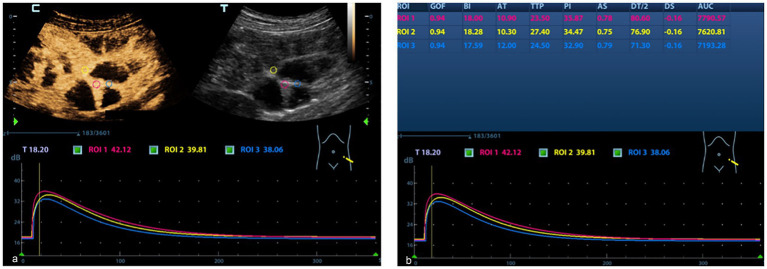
TIC of intestinal contrast enhancement with reflux obstruction type. (a) Bowel wall sampling site and corresponding TIC. The screen displays the contrast-enhanced image (C) alongside the conventional ultrasound image (T). (b) Plot of ROIs analyzed by dedicated software. The patient was a 30-year-old female with sepsis and volume overload. CEUS parameters were characterized by increased AUC and prolonged DT/2. These findings may reflect intestinal venous congestion and delayed contrast clearance, which may contribute to bowel wall edema and impaired perfusion.
